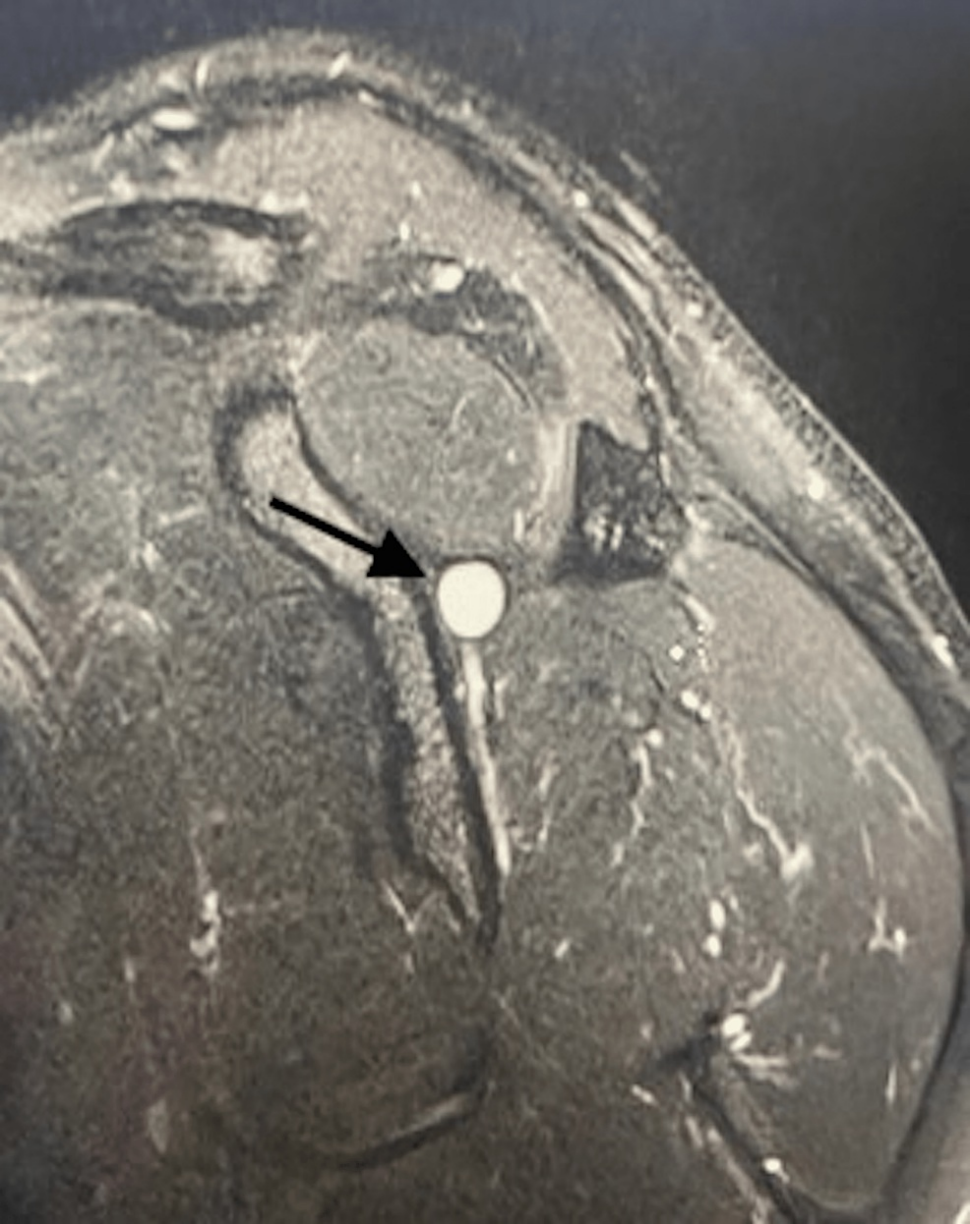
Sagittal MRI scan of the left shoulder showing the spinoglenoid notch ganglion cyst
